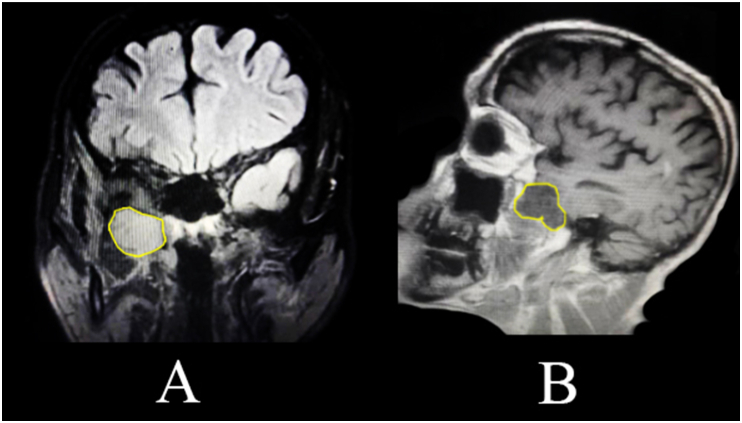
(A) The yellow circle shows a low signal mass in T1-Weighted MRI image with contrast. (B)The yellow circle shows a high signal mass in T2-Weighted MRI. (For interpretation of the references to colour in this figure legend, the reader is referred to the Web version of this article.)
